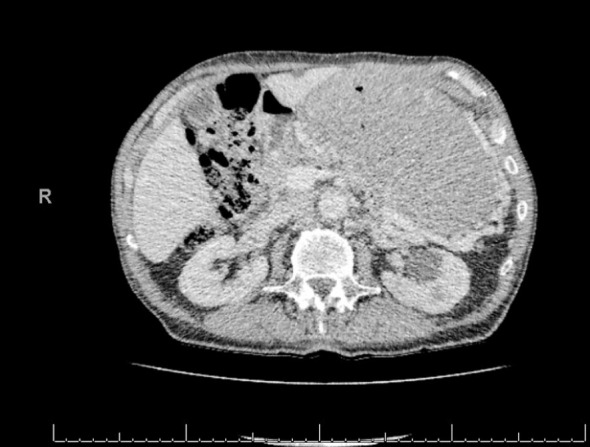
Computerised Tomogram. Transverse CT view, grossly enlarged stomach, pancreatic atrophy.
